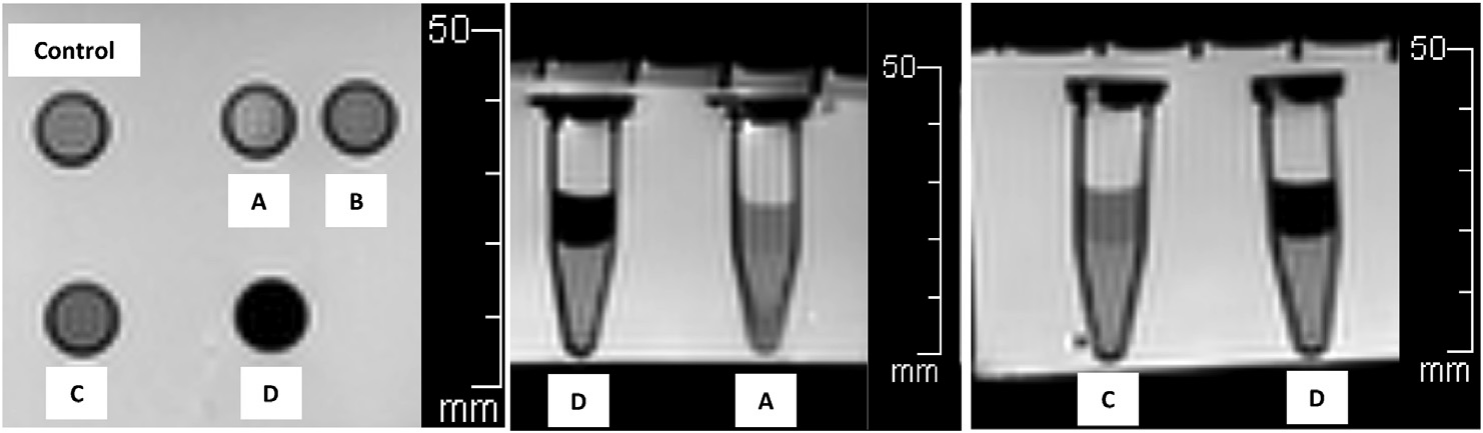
Axial view of IS-labeled ASCs from T2-weighted sequences of 3T MRI. Left: Axial view, Middle: Sagittal view, Right: Coronal view. A: 1 × 10^3^ cells/ml/microtube, B: 1 × 10^4^ cells/ml/microtube, C: 1 × 10^5^ cells/ml/microtube, D: 1 × 10^6^ cells/ml/microtube.
